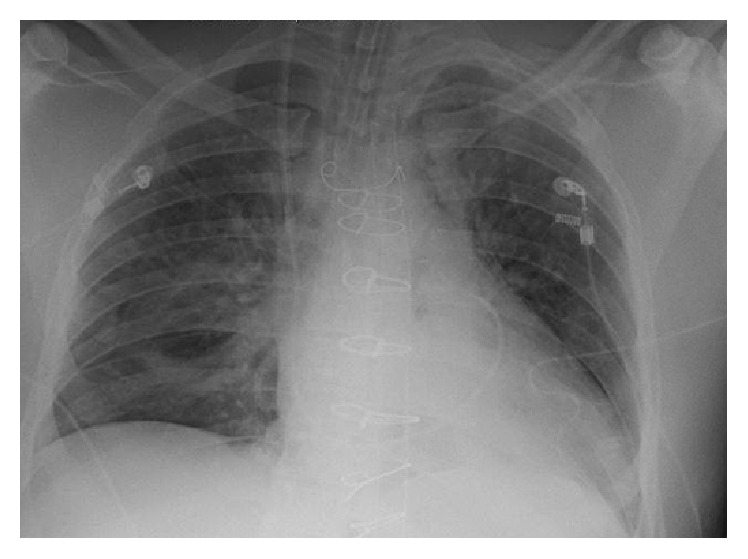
Chest X-ray: clear lungs, cardiomegaly, and wide mediastinum.
